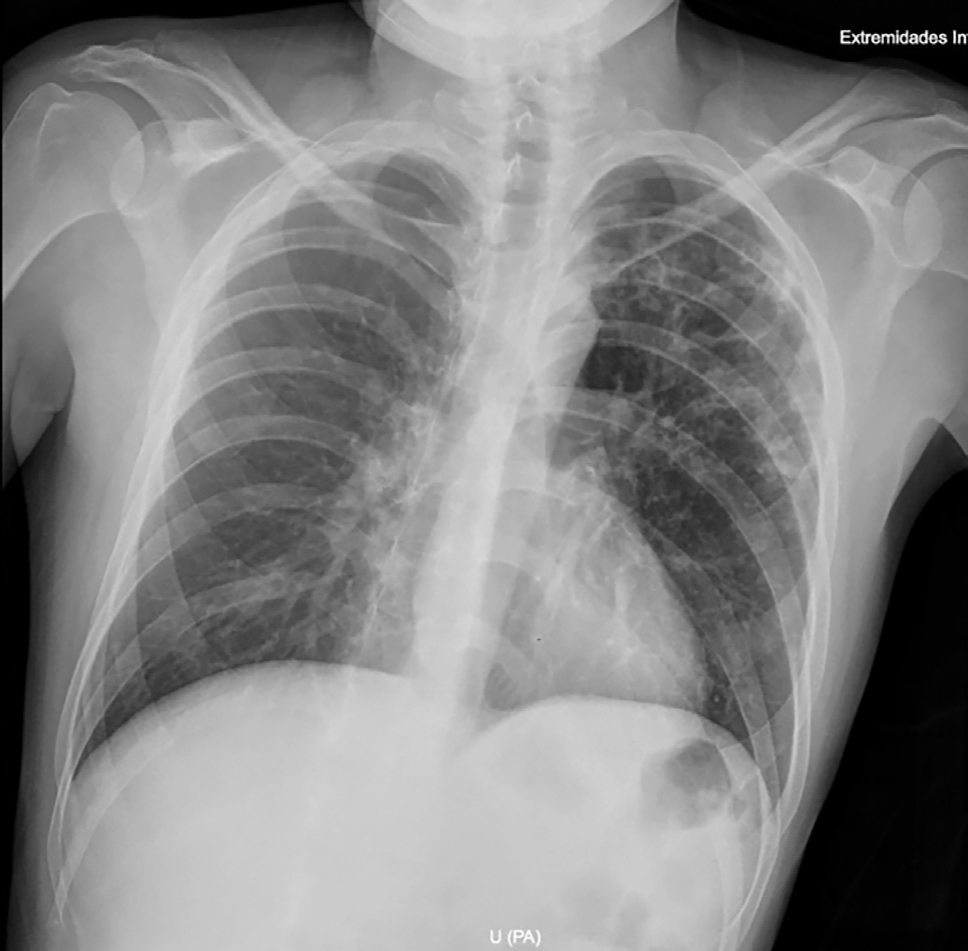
Chest X-ray showed cavitation in the upper left region and nearby smaller ones.
